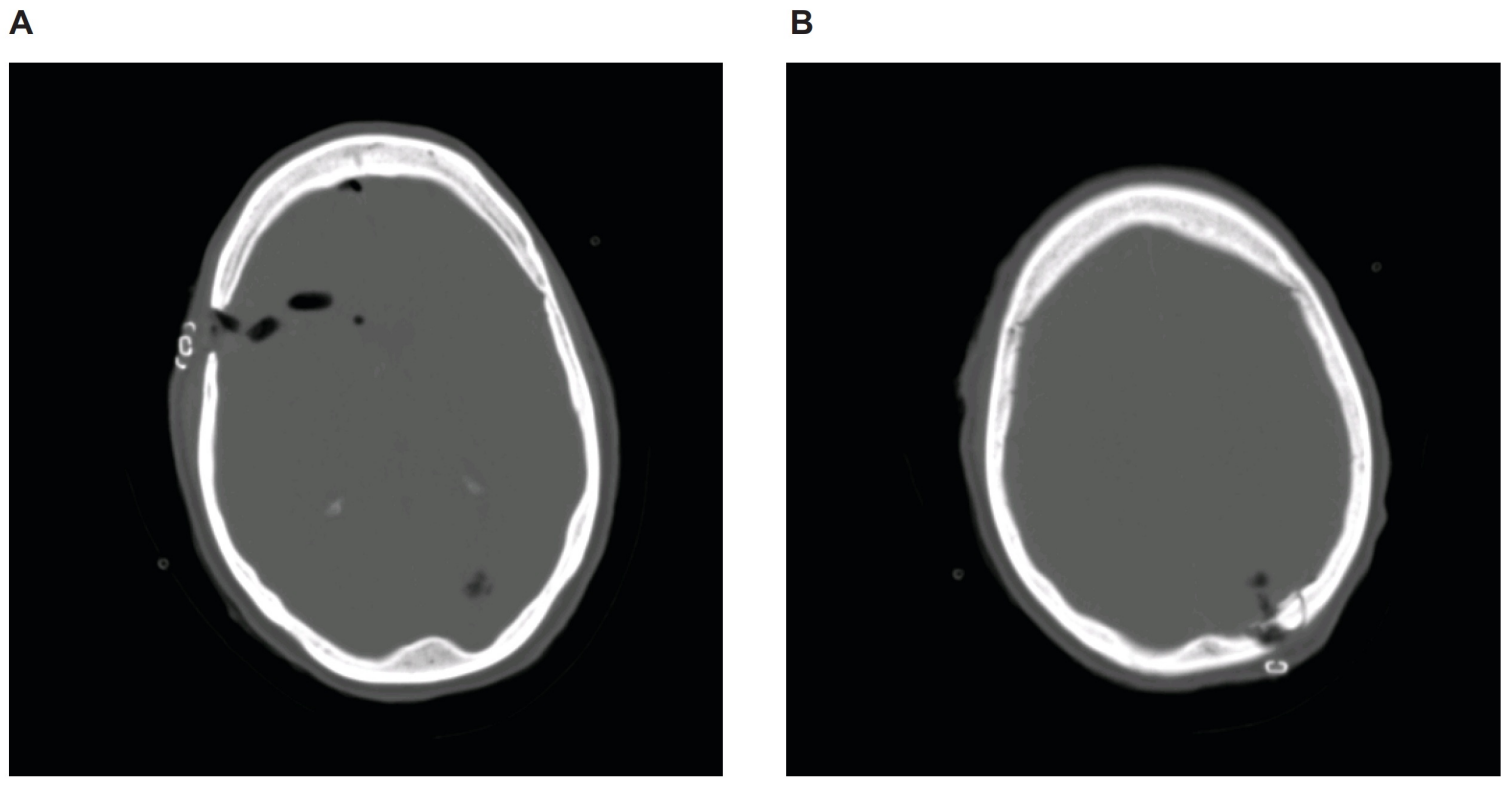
CT study of the head of the gunshot wound on postoperative Day 1 (A) Image shows the removal of bone fragments in the right frontal region with mild pneumocephalus. (B) Image shows resection of the bullet fragment in the left parietal region. Both panels are shown in the bone window.
